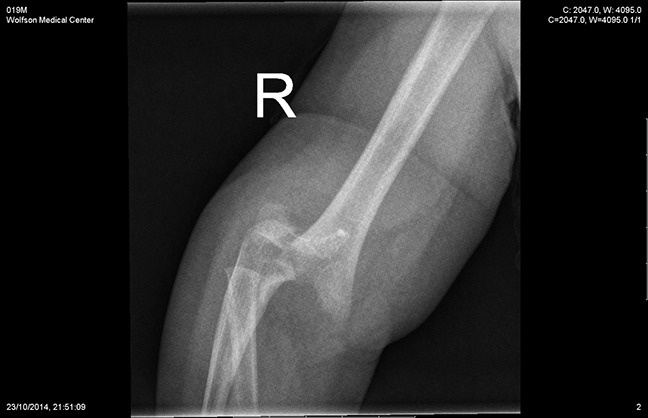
The initial radiograph of the fracture
